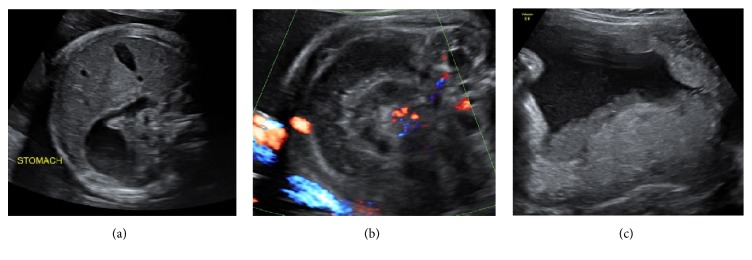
Ultrasound images of the key antenatal findings: (a) dilated appearing stomach with relatively normal duodenal diameter, not consistent with duodenal atresia; (b) concentric small bowel visible around the twisted mesenteric pedicle (whirlpool sign) and the superior mesenteric vein malpositioned on the left of the artery; and (c) dense sediment noted in the amniotic fluid, which was noted to be bile at the time of delivery.
